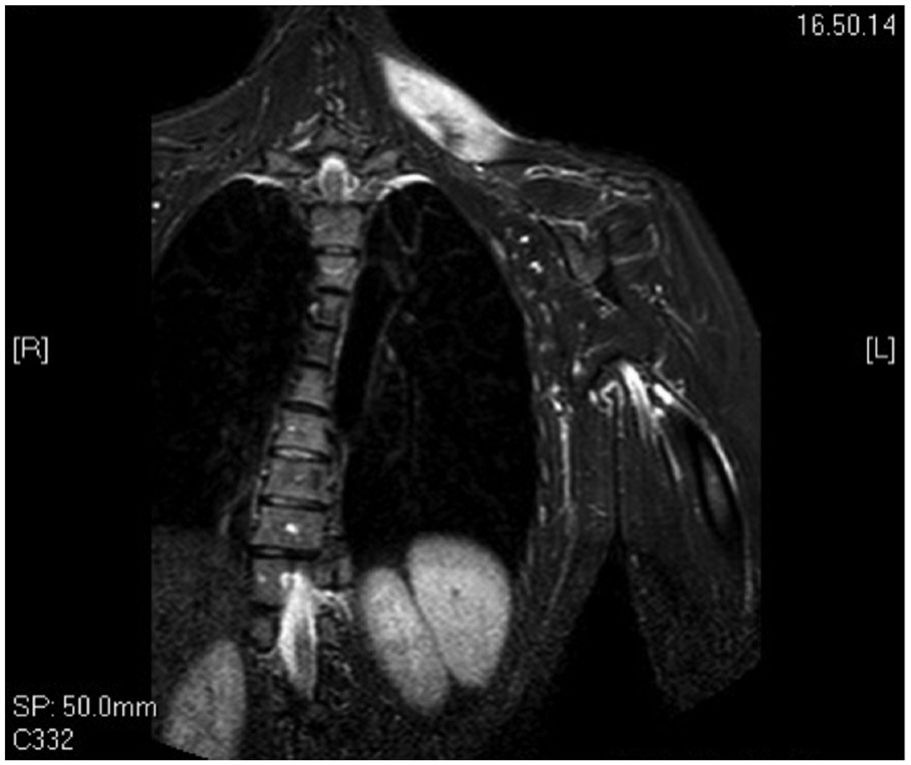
Pre-operative MRI shows the mass in the upper third of the trapezius.
